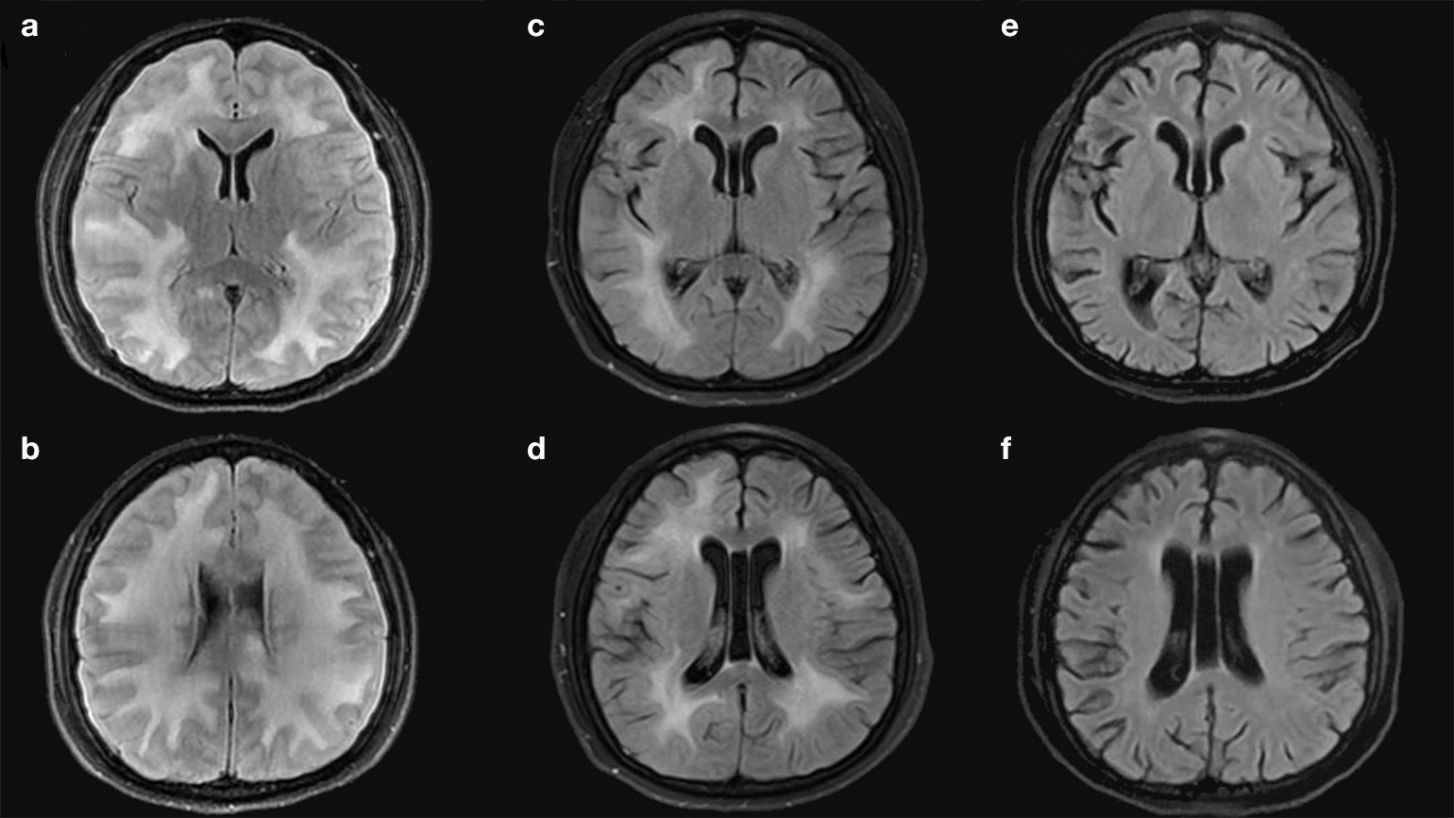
(a–f) MRI done in different periods.
